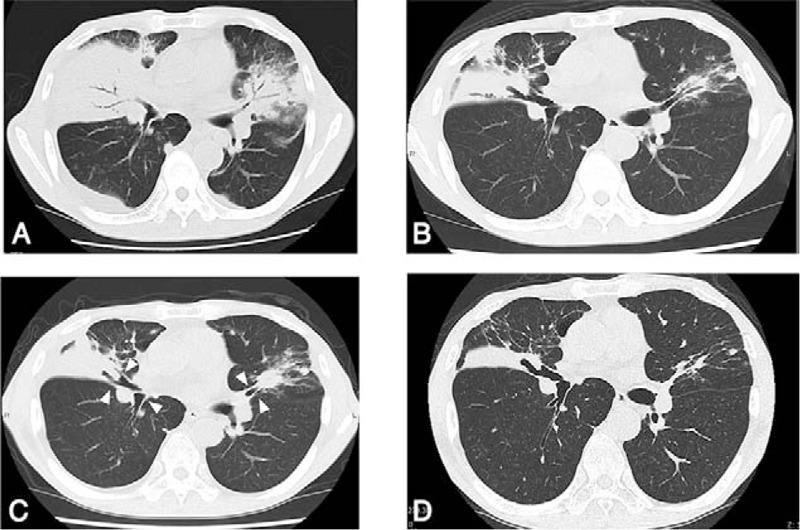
Computed tomography of the chest. (A) At the diagnosis. (B) One month later from the initiation of remission induction therapy. (C) At the time of relapse. (D) At 5 weeks after the initiation of re-remission induction therapy. The white arrowheads indicate the exacerbation of bronchial wall thickness.
